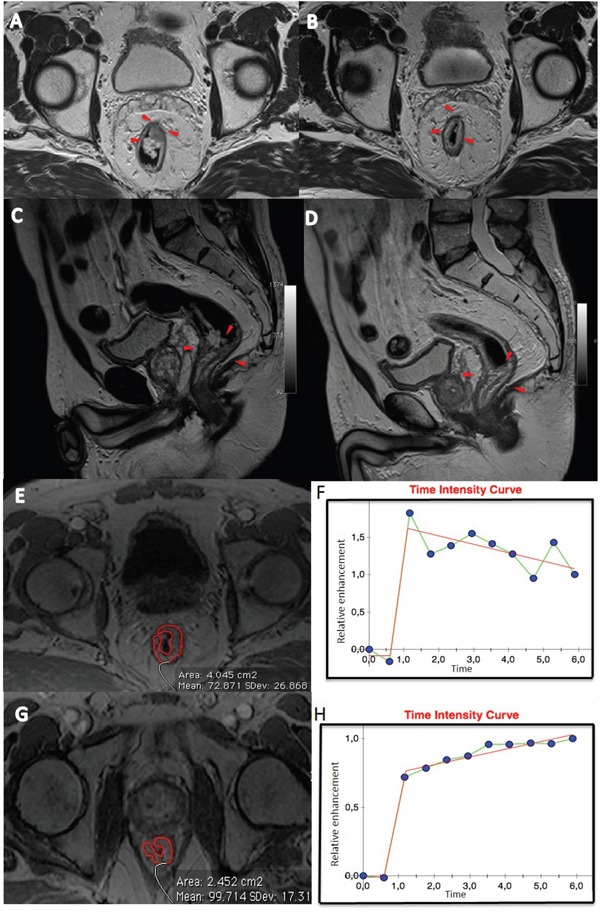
Patient n. 64: T2-weighted images in axial A and sagittal C plane before B and after treatment D. The morphologic images (A and C) before CRT, showed heterogeneous irregular thickening along the rectal wall spreading into the perirectal fat (A, arrowheads). After CRT, a hypointense area relating to rectal wall thickening is still visible (B and D, arrowheads). Median Time intensity curve of volume of interest (E and G), segmented by expert radiologist, before treatment is shown in F and after treatment in H. These curves showed different contrast enhancement, with a ΔSIS of 31.93% classifying the patient as responder.
